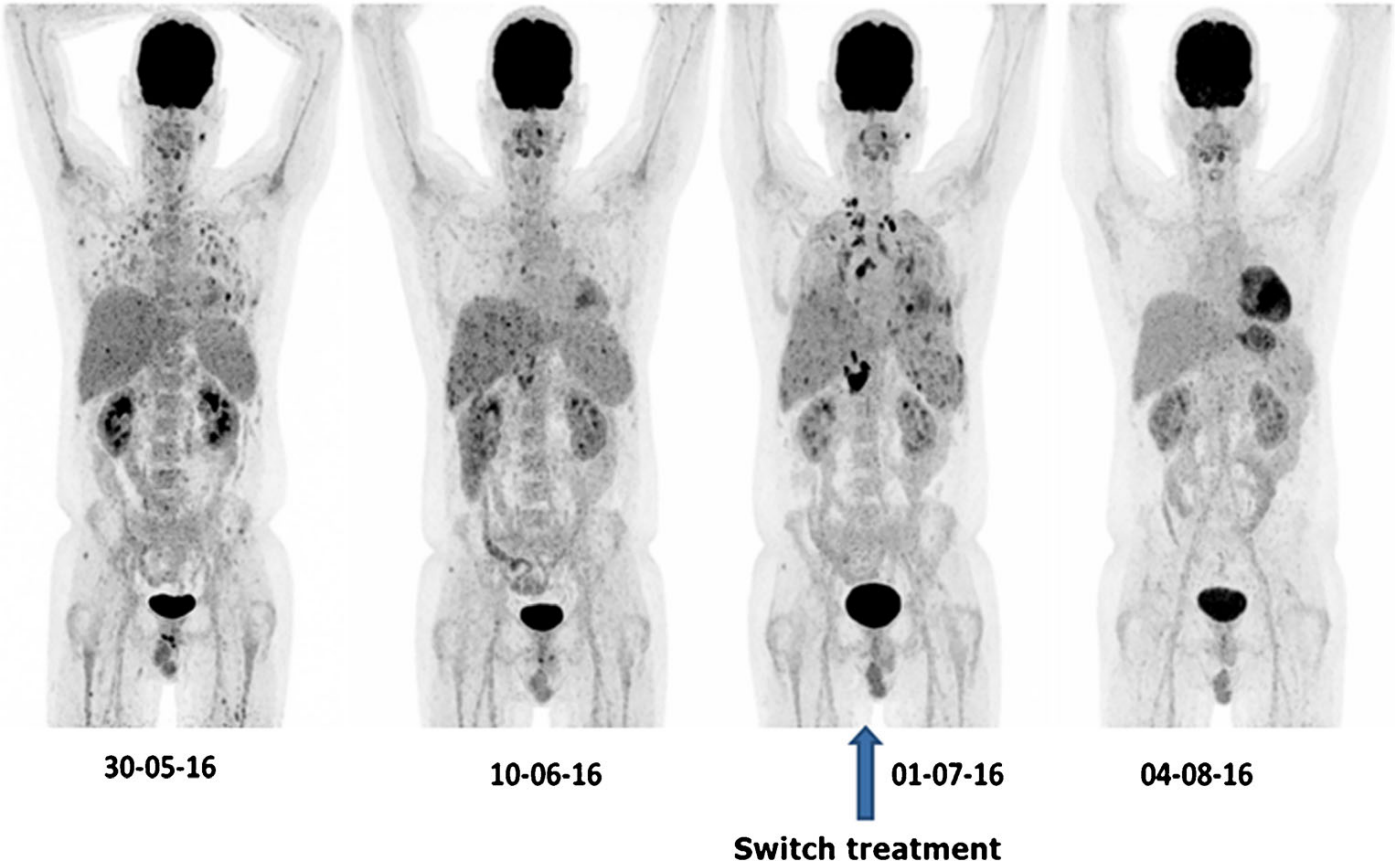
FDG-PET MIP images of a 38-year-old male with acute lymphoblastic leukemia (ALL) who was first thought to have a bacterial infection but was unresponsive to antibiotics. A clinical diagnosis of invasive candidiasis was made, at baseline FDG-PET/CT a global TLG of 401. Follow-up FDG-PET/CT showed a good response with a TLG of 30. Then the patient developed fever, with negative blood cultures, and a repeated FDG-PET/CT showed new lesions with a global TLG of 900. The antifungal therapy was modified, and the patient had a complete metabolic response at the last scan
